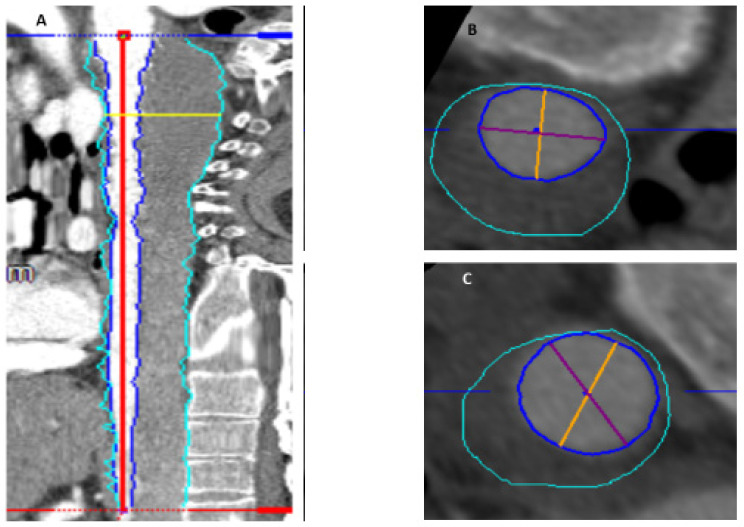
Thrombus volume measurements before and after the FET/CET procedure using a dedicated software. (A) Centerline in the true lumen, thrombus area has been marked and measured manually for each CT slice (grey area), separated from the true lumen. (B,C) Volume of the thrombus was measured by subtracting the true lumen from total aortic diameter.
